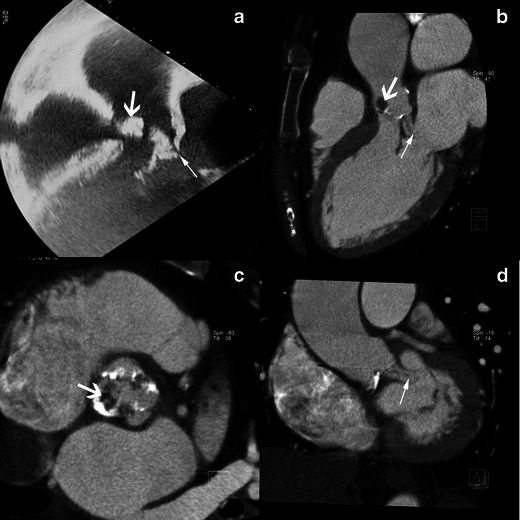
Results of echocardiography (TEE) and MSCT studies in a case of aortic valve infective endocarditis complicated by a fistula between aorta and left atrium. The TEE study is shown in the 120-degree long-axis view (a) and the MSCT acquisition with MPR reconstructions in the LVOT view (b), the aortic valve plane view (c), the coronal oblique view at the level of the aortic root (d) show a fistula between aortic root and the left atrium (*thin white arrow*) and a large vegetation on the aortic valve (*thick white arrow*)
